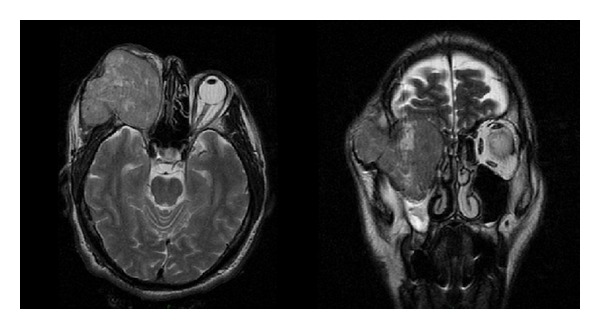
Axial and coronal MRI image showing a right intraconic and extrasonic mass with ocular globe displacement and temporal muscle and zygomatic bone infiltration.
